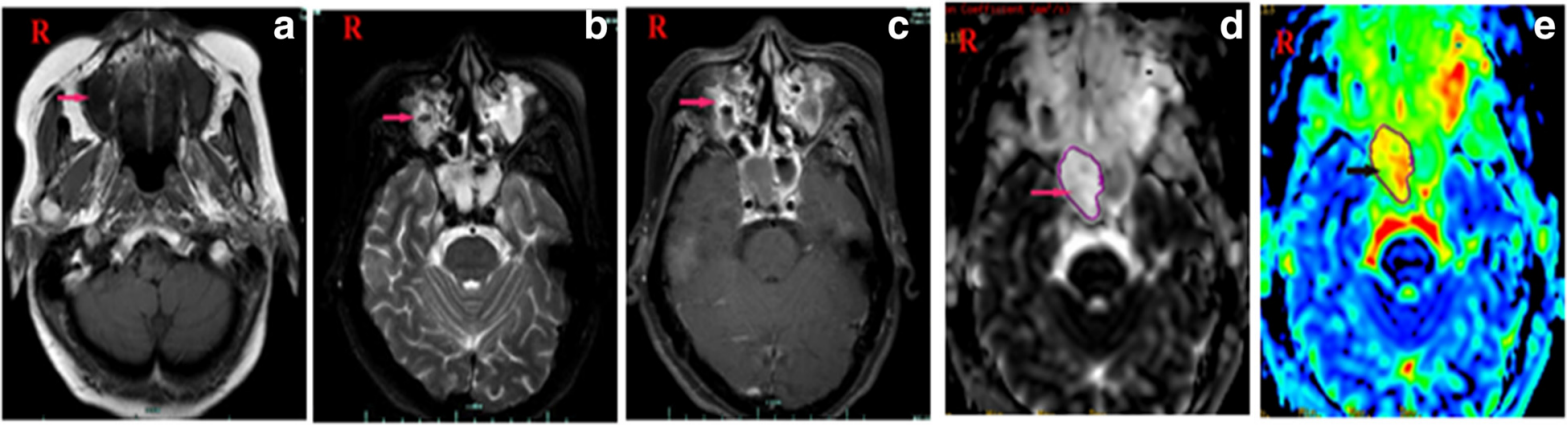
MRI of Case 10 showingan abnormal signal in the right maxillary sinus and right nasal cavity. a) TheT1-weighted signals b) and the T2-weighted signals were hyperintense c) and the contrast-enhanced T1-weighted MRI images show strong enhancement. d) DWI suggested hyperintense lesions were in the right maxillary sinus and right nasal cavity. e) The corresponding ADC map reveals (ADC = 1.78 × 10^-3^ mm^2^/s).
